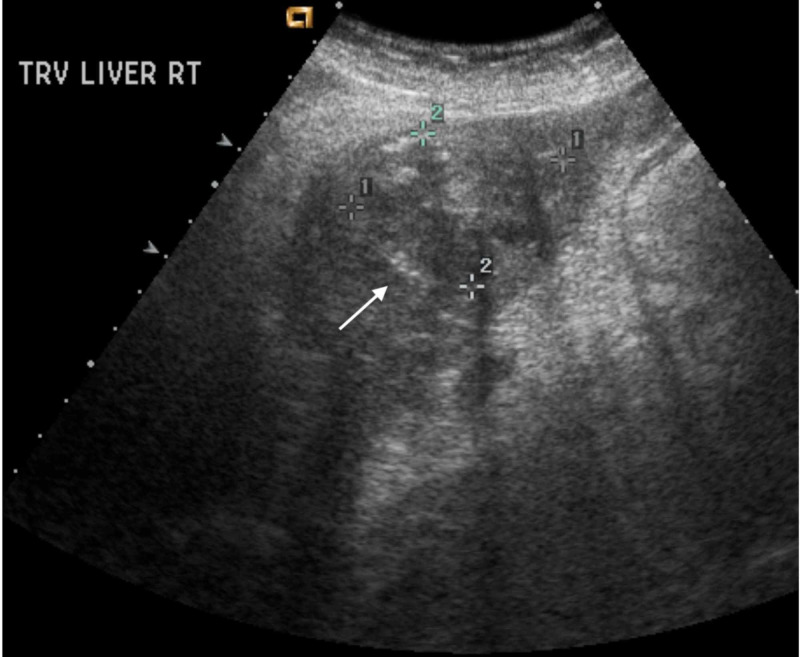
Liver Ultrasound Liver Ultrasound revealed a 4.9 cm x 3.6 cm x 4.2 cm mass in the left lobe with a small amount of perihepatic ascites.
